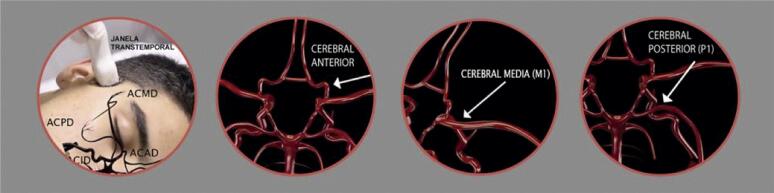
Doppler transcraniano e janela ultrassonográfica transtemporal (11A); representações esquemáticas de artérias cerebrais anterior (11B), média (11C) e posterior (11D).c) Janela transforaminal: única via de acesso aos fluxos em luzes de segmentos intracranianos das vertebrais (V4) e à origem da basilar (Figura 12), além das artérias cerebelares posteroinferiores (ramos cruciais como via colateral de escoamento de fluxo em casos de oclusão de vertebral acima da emergência dos mesmos).Figura 12 f12:
